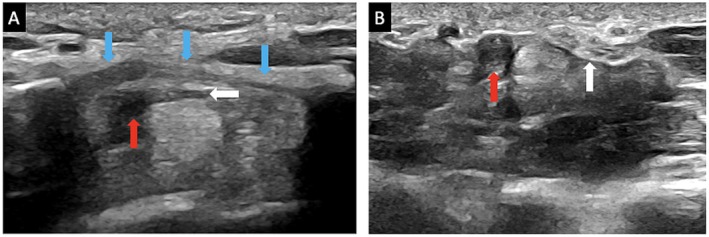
Sequential transverse gray‐scale ultrasound images showing persistent median artery pseudoaneurysm with thrombosis at (A) and just distal (B) to the level of the carpal tunnel. Images demonstrate a hypoechoic tubular mass (red arrows) adjacent to the compressed median nerve (white arrows), flexor tendons, and transverse carpal ligament (blue arrows).
